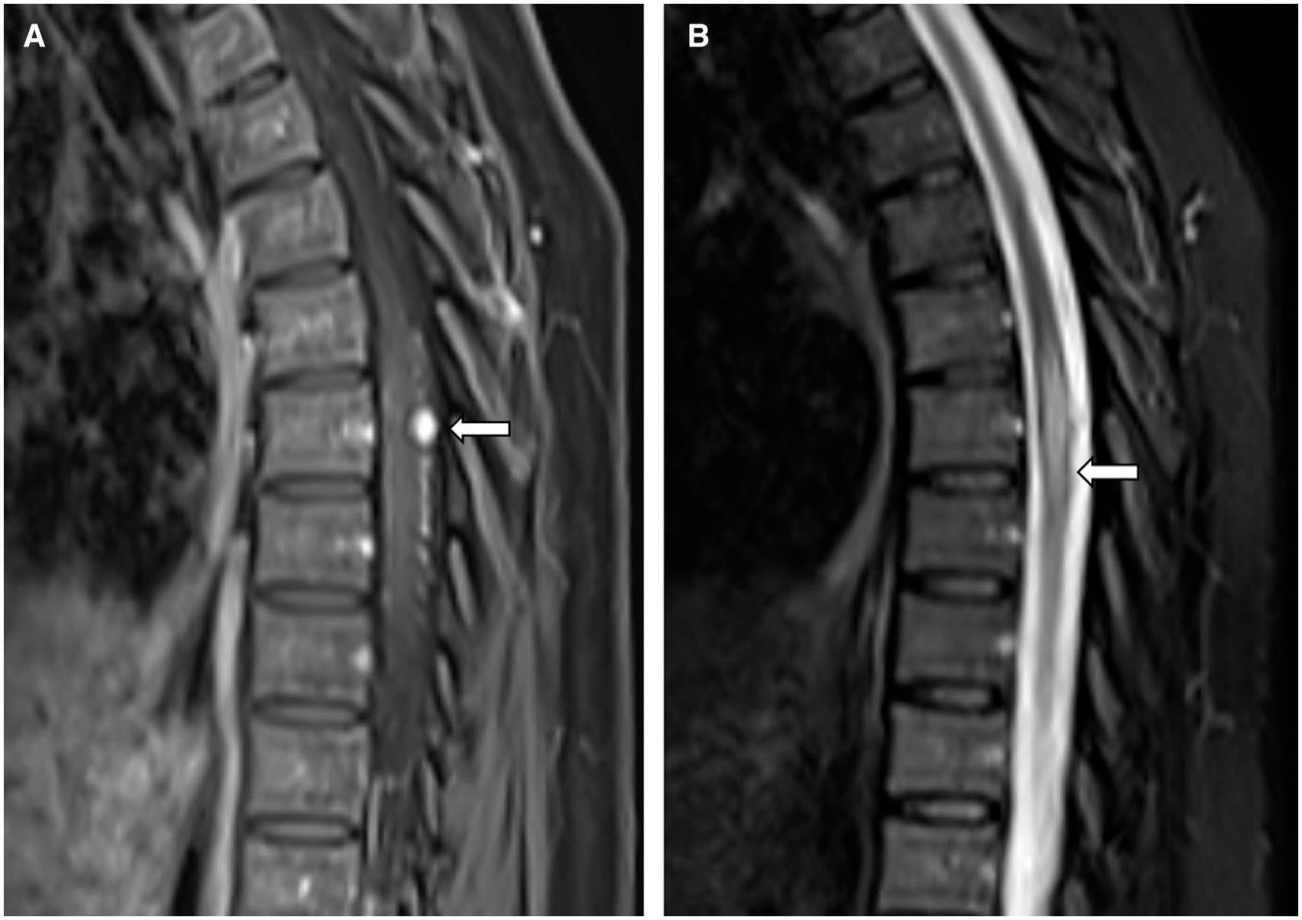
Medular haemangioblastoma. (A) Axial contrast-enhanced T1-weighted MR image of the dorsal spine shows intramedular focal lesions with avid enhancement in T7 (arrow). (B) Axial T2-weighted MR image shows perilesional spinal cord edema (arrow).
